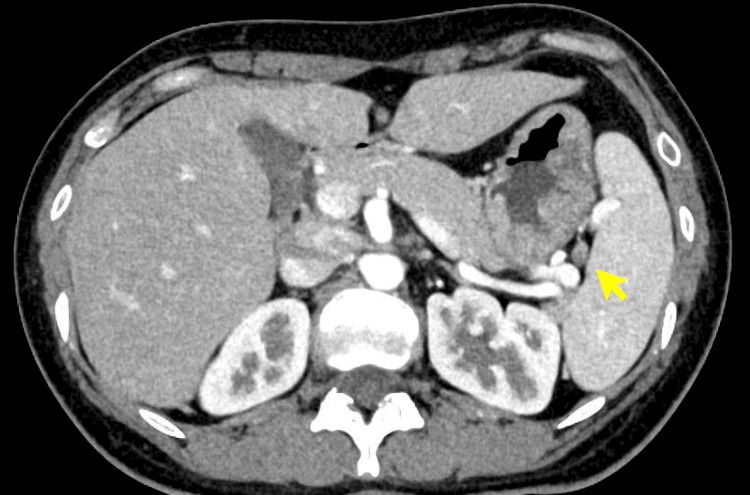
Axial contrast-enhanced CT scan Enlarged splenic hilar (#10) lymph node (yellow arrow).
